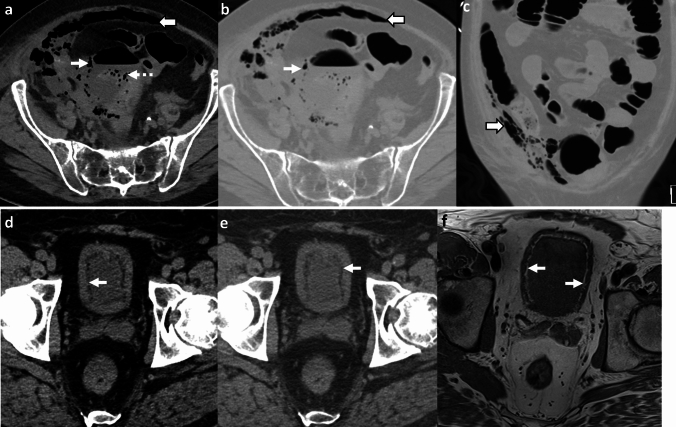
Pitfalls for emphysematous cystitis. a–c Urinary bladder injury. A 73-year-old with a newly diagnosed bladder tumor, post-transurethral resection of bladder tumor with pain and hematuria after the procedure. CT images show air in the right lateral bladder wall (solid white arrow, a, b), air within the tumor along the left lateral wall (dotted white arrow, a), and extensive extraperitoneal air (block white arrows, a–c). Findings are consistent with extraperitoneal bladder rupture. d–f Submucosal fat. A 54-year-old male patient with colon cancer underwent CT for staging. CT images show a low-density stripe along the anterior and lateral bladder walls (solid white arrows, d, e). T1-weighted (TIW) non-fat-saturated axial MRI image shows a hyperintense submucosal stripe (solid white arrows, f) which suppressed on fat-saturated imaging (not shown), confirming the presence of submucosal fat
